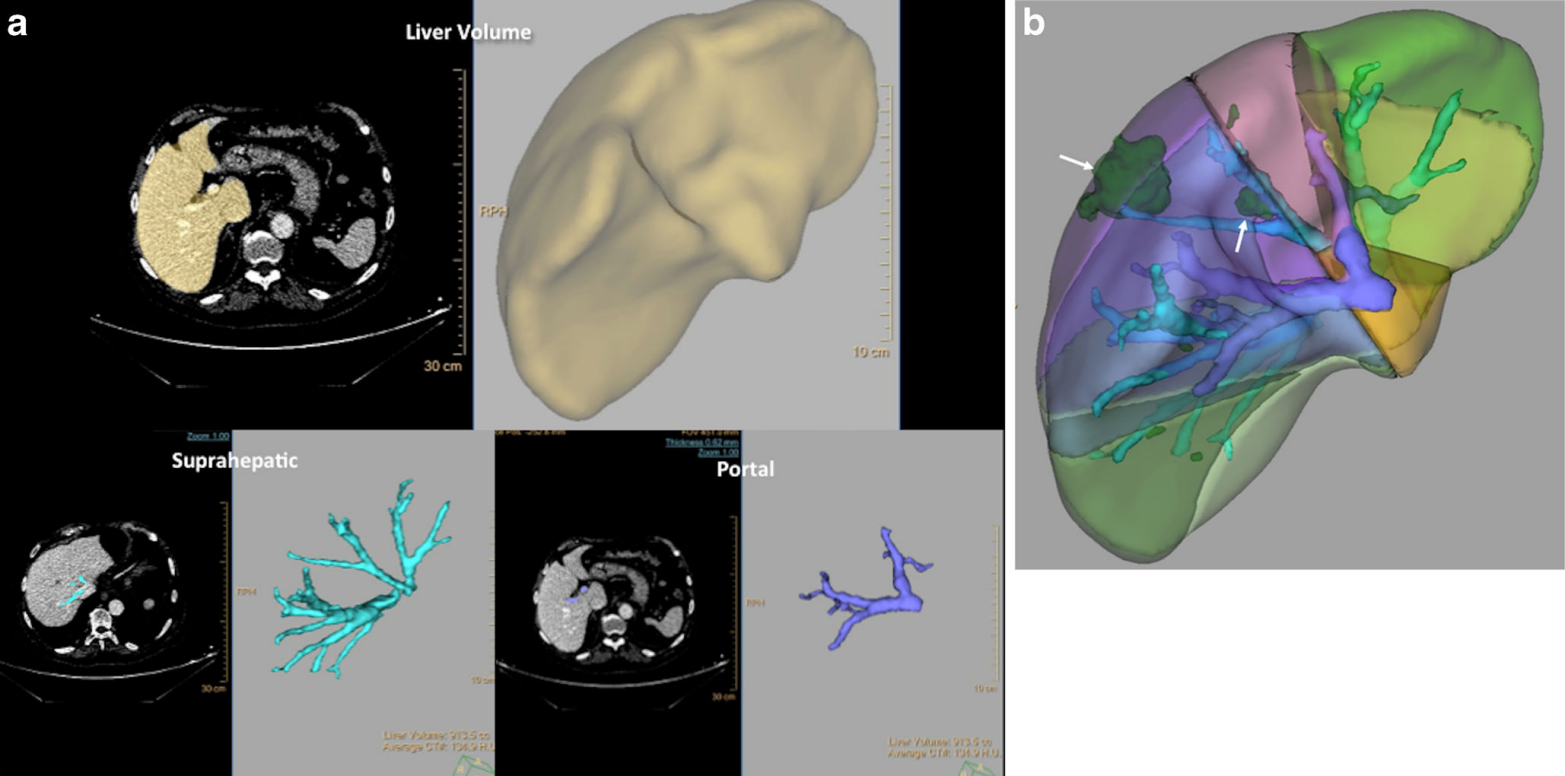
CT volumetry in a patient with colorectal cancer liver metastases. Liver area determined with automated method (yellow) (top-left). Total liver volumetry (1185 cc) (top) and images of the segmentation of the suprahepatic and portal vessels (a). Automatic Couinaud liver segmentation using CT images (b), which allows the location of the metastatic deposits (green – white arrows)
